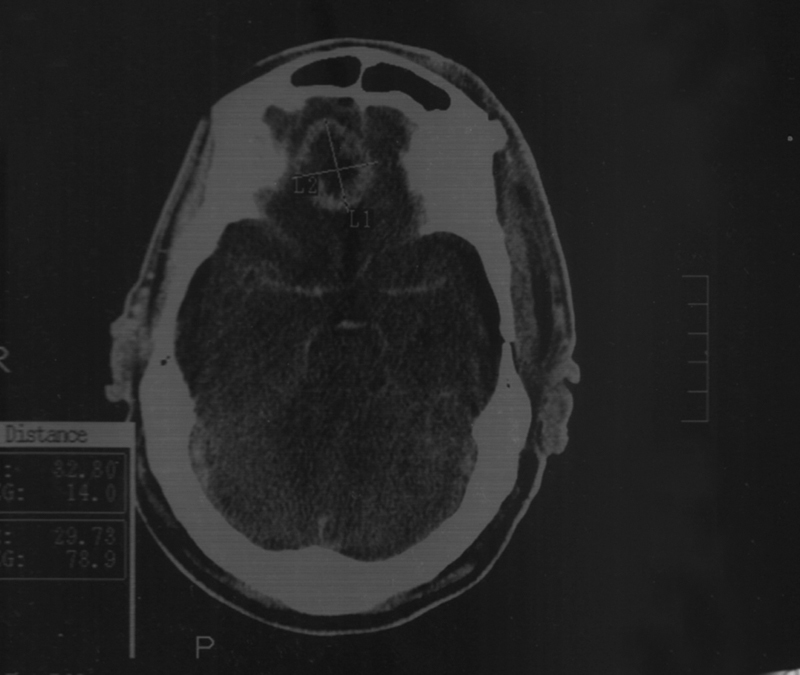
Postcontrast computed tomography (CT) scan, showing an intra-axial right frontal space-occupying lesion.
